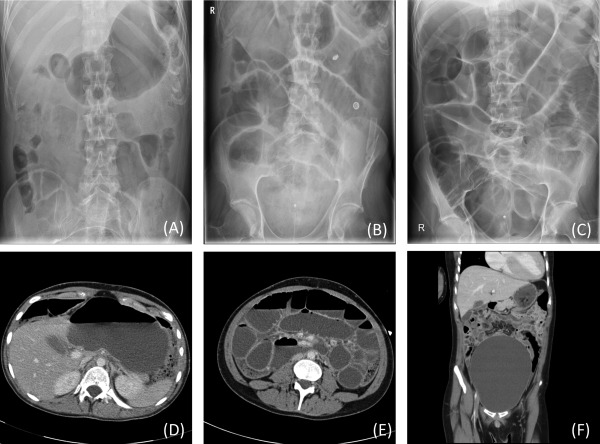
Radiological imaging studies. Abdominal radiograph (A–C) showed an evolution of intestinal pseudo‐obstruction involving both small and large bowels (radiograph performed on admission, day 5, and 10, respectively); computed tomography abdomen and pelvis (D) and (E) showed dilated, fluid‐filled stomach and small bowel loops; (F) very distended bladder and fecalization of small bowel.
